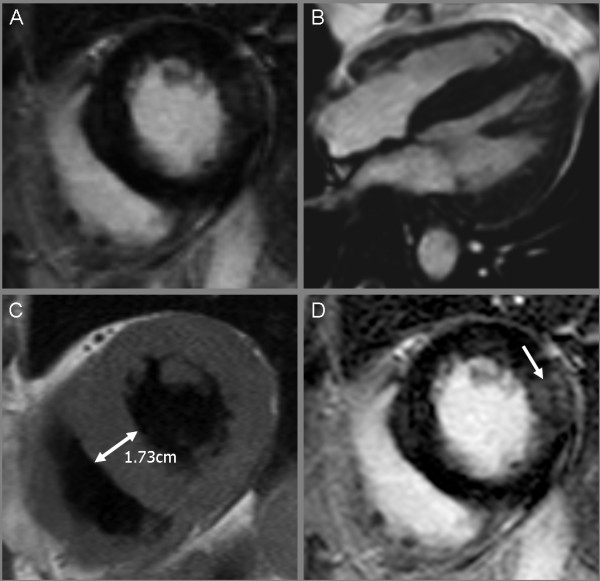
Cardiac MRI showing a marked hypertrophic cardiomyopathy. Balanced Fast Field Echo (bFFE) cine sequence, short axis view (A); 4 chamber view (B); a left ventricular hypertrophy with normal contractility was recognized. T1 weighted black blood Turbo spin echo sequence, short axis view (C); left ventricular hypertrophy is evident, specifically in the septal region. Corresponding view with transmural late Gadolinium enhancement (arrow) in the lateral wall using a T1 weighted inversion recovery 3D fast gradient echo sequence (D).
